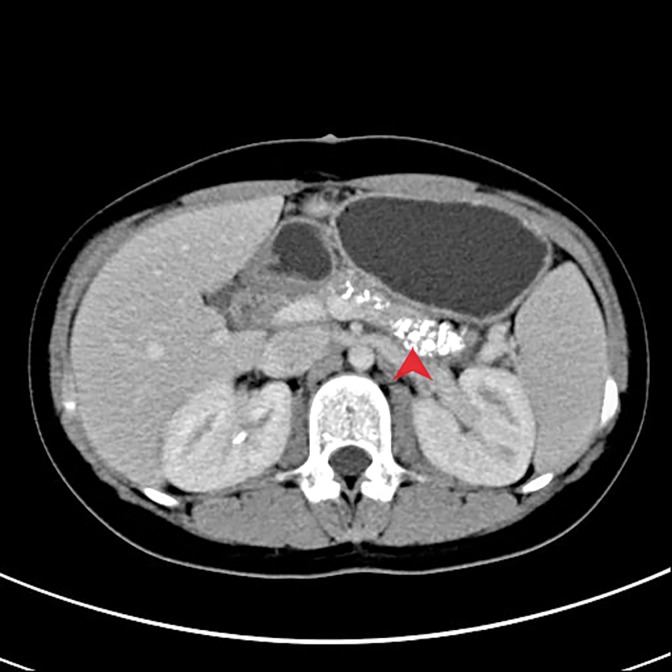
Seventh month follow-up. Abdominal CT showing parenchyma calcification. Red arrow: pancreatic calcification.
